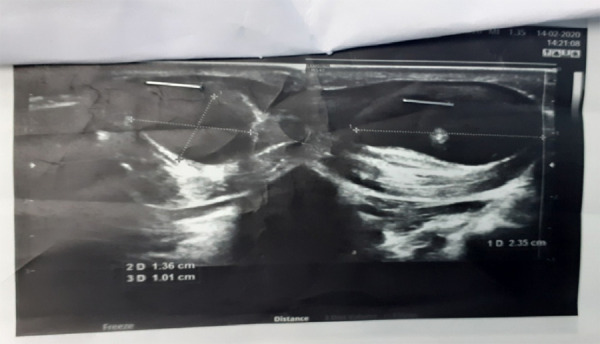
Ultrasound findings showing defined cyst measuring 1.5×2.5×1.0 cm within thenar muscle with intracystic echogenic contents and tiny cysts.
